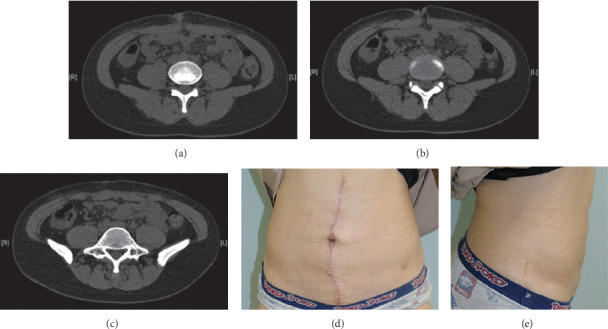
(a–c) Postoperative abdominal computed tomography. (a) 3 cm above the superior border of the umbilicus; (b) center of the umbilicus; (c) 2 cm below the inferior border of the umbilicus. There is no apparent recurrence of the hernia. (d-e) Appearance three months after surgery. The patient's abdominal bulge has resolved.
